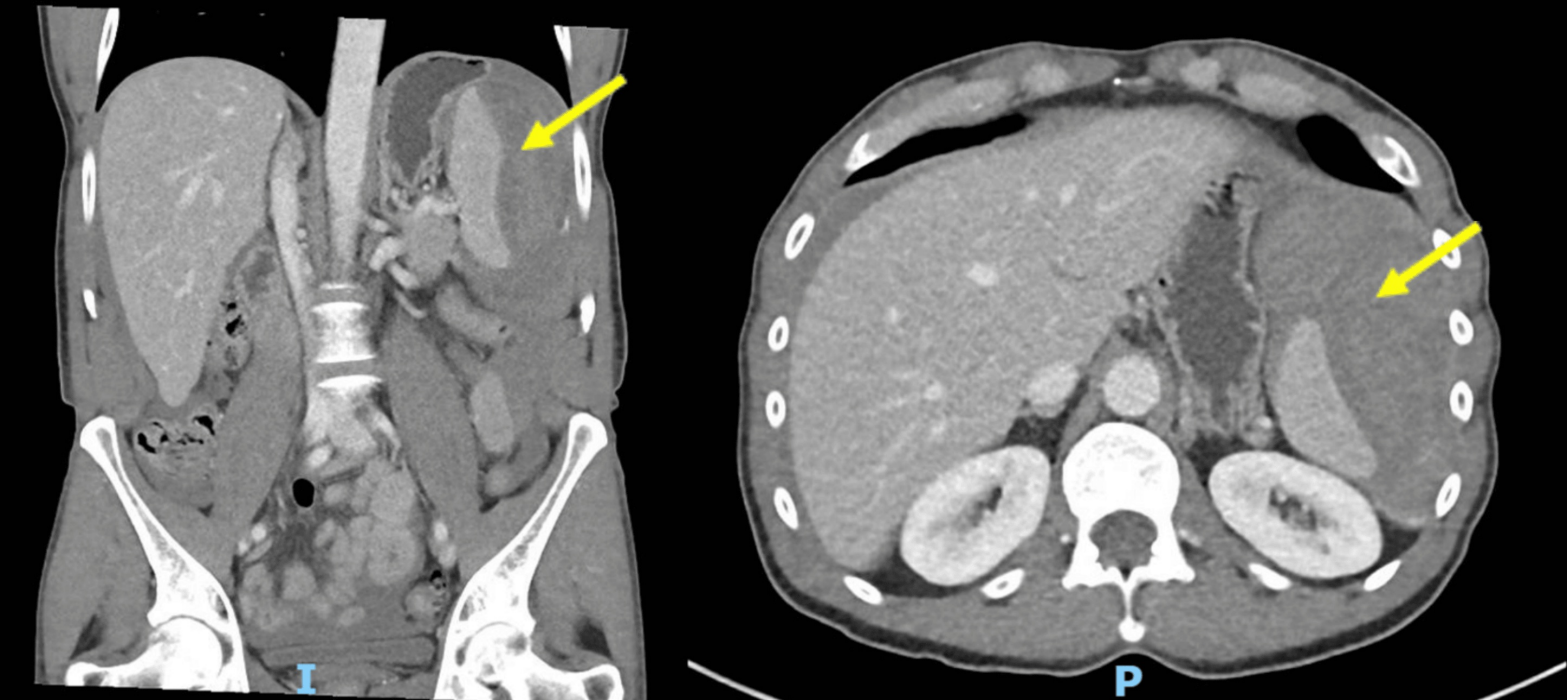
CT abdomen and pelvis demonstrating a large perisplenic hematoma (yellow arrow) consistent with splenic rupture. Coronal section on the left; axial section on the right. CT, computed tomography
